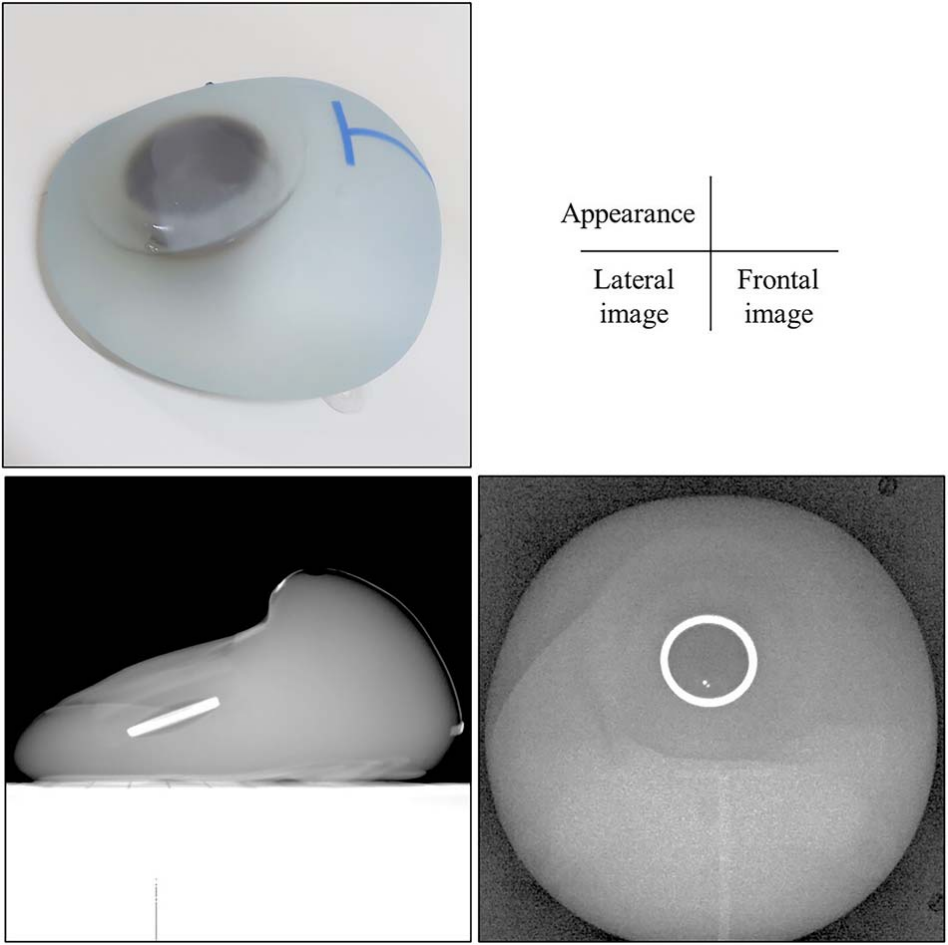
The appearance and X-ray images of Flora TE.
